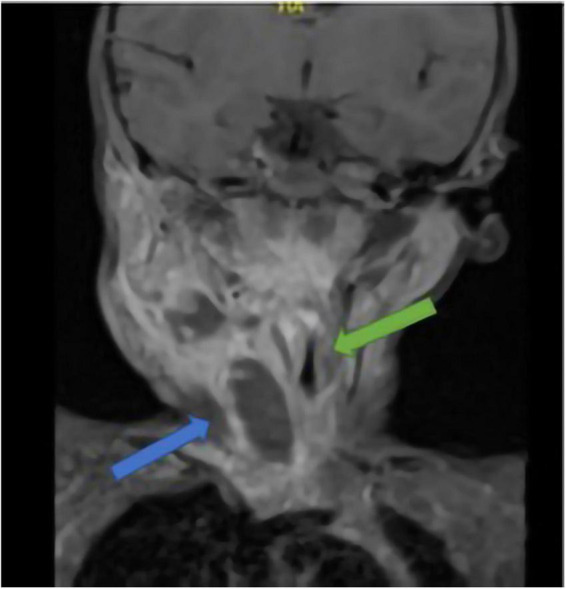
Cervical MRI of case 2; blue arrow: abscess; green arrow: trachea stenosis.
